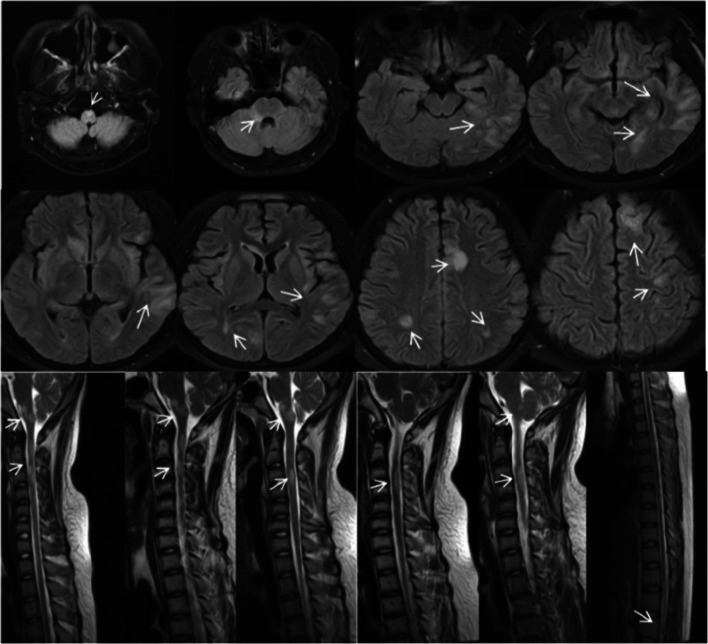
case 1: Brain MRI showed lesions in the bilateral frontal, apical, temporal, occipital cerebral gyrus, bilateral insular, right brachium pontis, medulla, and corpus callosum on Flair after progression of the disease. Spinal MRI showed lesions in the medulla oblongata, C2-T1, T4, T7, T11- T12, and spinal conus on T2 after progression of the disease
